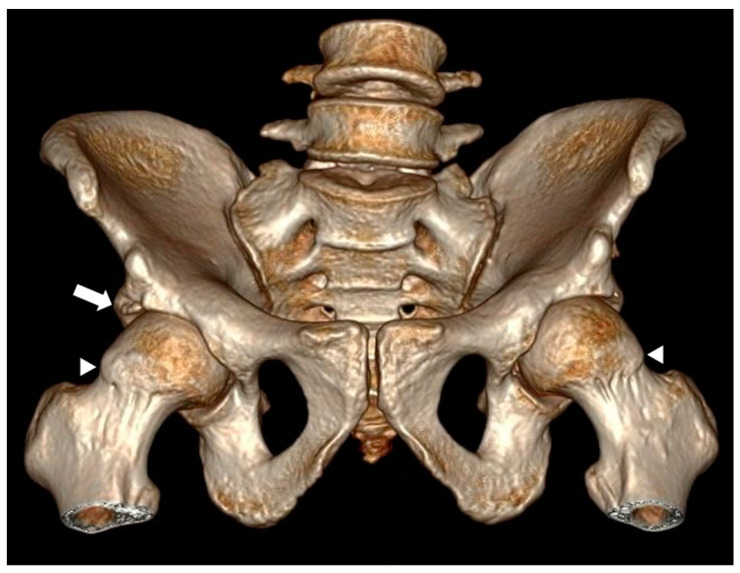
Computed tomography image with 3D volumetric reconstruction showing anterosuperior bony prominence in the cervical–cephalic transition of the bilateral femur, as well as bone excrescence in the right acetabular roof. Findings in relation to right mixed-type and left cam-type femoroacetabular impingement are marked with white arrowheads.
